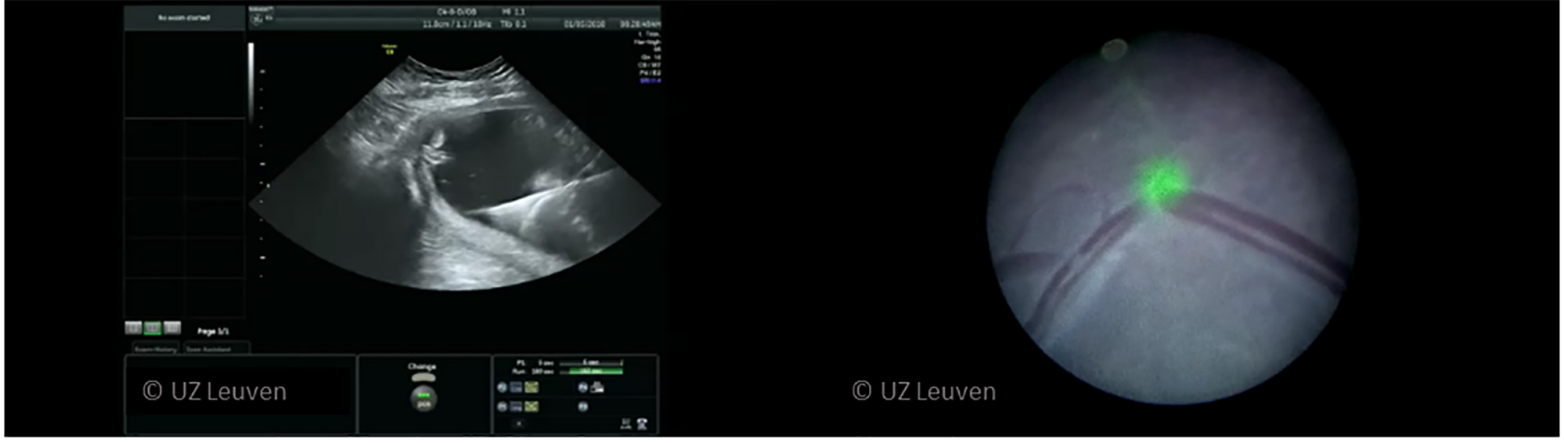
Typical view with ultrasound (left) and fetoscopically (right) during active lasering. Reproduced with permission and copyright: UZ Leuven, Belgium.
